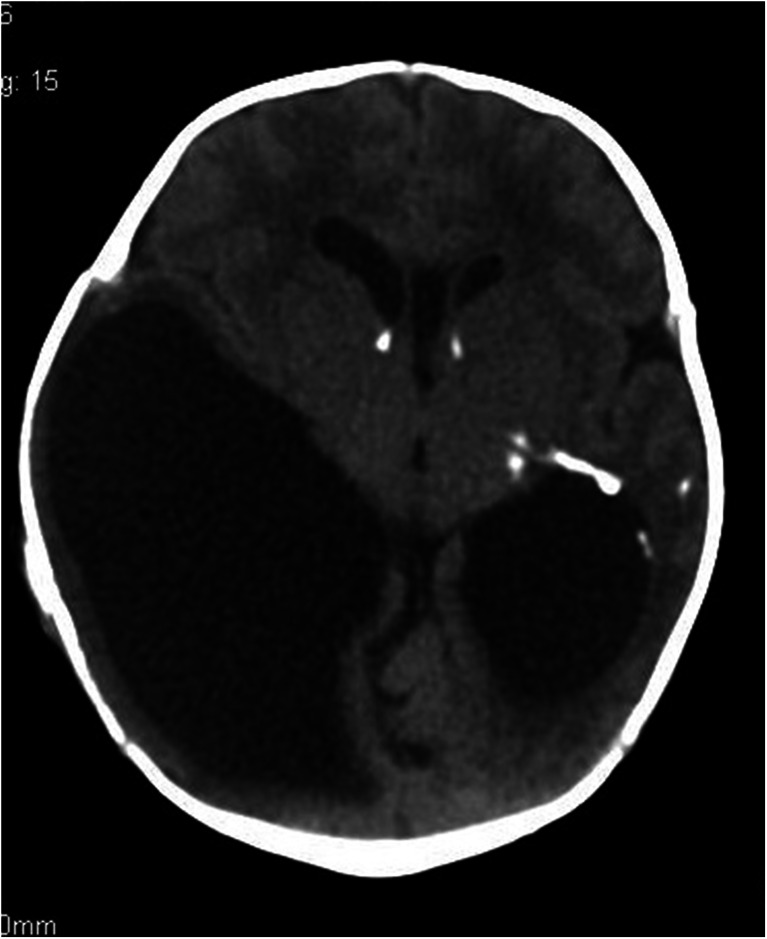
Massive bilateral distension of the occipital and temporal horns of the lateral ventricles of the brain. Periventricular calcifications. Incomplete brain myelination. Nonenhanced CT scan
